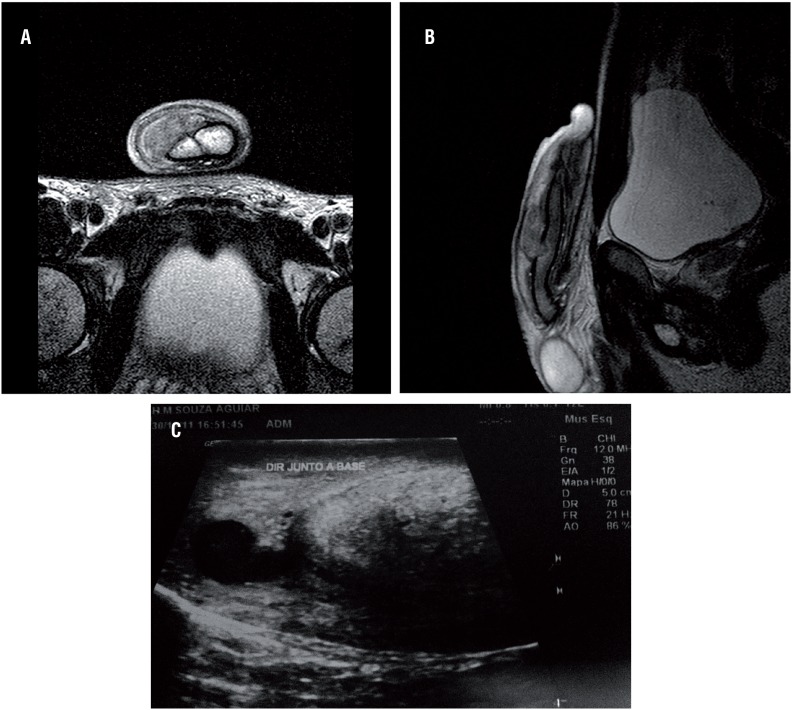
Patient with doubtful clinical picture of PF submitted to penile MRI demonstrating right corpus cavernosum base rupture with moderate hematoma in axial and sagittal images (A+B). Ultrasound demonstrating right corpus cavernosum base rupture with mild hematoma in another patient with doubtful clinical picture of PF (C).
